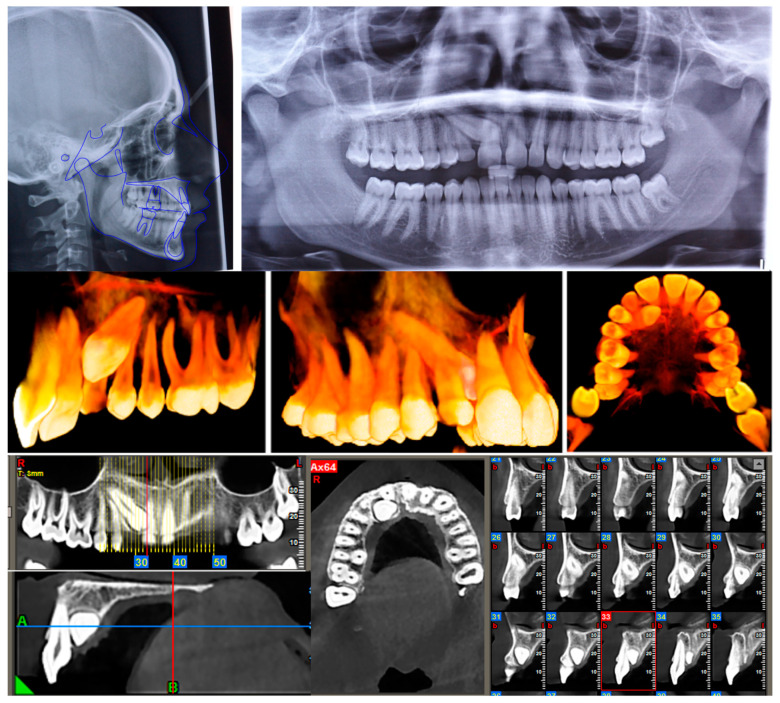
Pretreatment OPT, cephalometric analysis, and CBCT.
